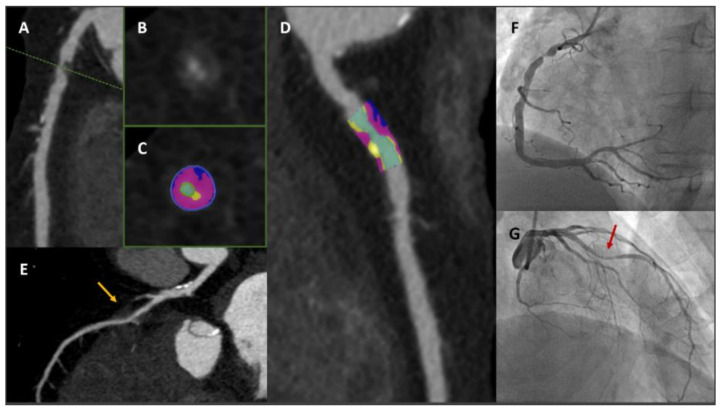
A 75-year-old male with diabetes and dyslipidaemia developed typical chest pain and cCTA was prescribed. Panels (A–D): critical stenosis with high-risk plaque features at proximal RCA. Panels (B,C): cross-section images at most stenotic level (green dotted line). Panels (C) (short axis) and (D) (long axis) with advanced plaque analysis: residual lumen identified with colour green, fibrofatty component (<30 HU) identified with colour blue, fibrotic component (between 30 and 350 HU) identified with colour purple, calcific component (>350 HU) identified with colour yellow. Panel (E): LAD characterised by non-significant calcific disease at proximal segment and deep myocardial bridge at mid segment (yellow arrow). Panels (F,G): ICA showing critical stenosis at proximal RCA (Panel (F)) and deep myocardial bridge at mid LAD (Panel (G), red arrow). *cCTA: cardiac computed tomography angiography; RCA: right coronary artery; HU: Hounsfield unit; LAD: left anterior descending; ICA: invasive coronary angiography.*
